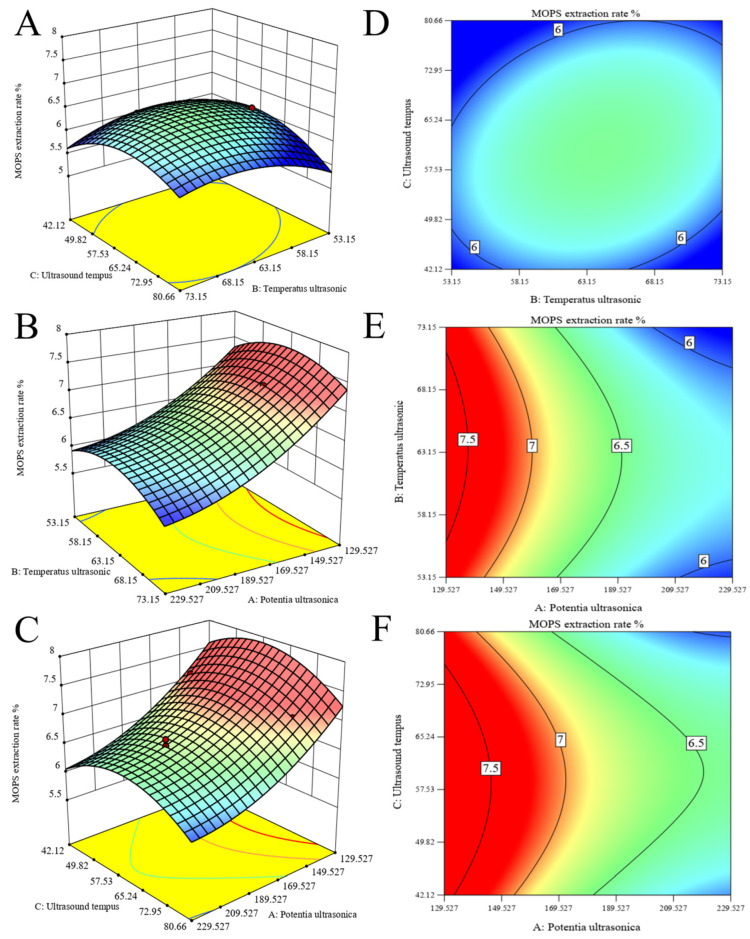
Optimization of MOPS extraction. Response surface plots (A–C) and contour lines (D–F) of ultrasonic extraction interactions were obtained, and extraction temperature, extraction time, and ultrasonic power were used as independent variables to optimize polysaccharide extraction.
